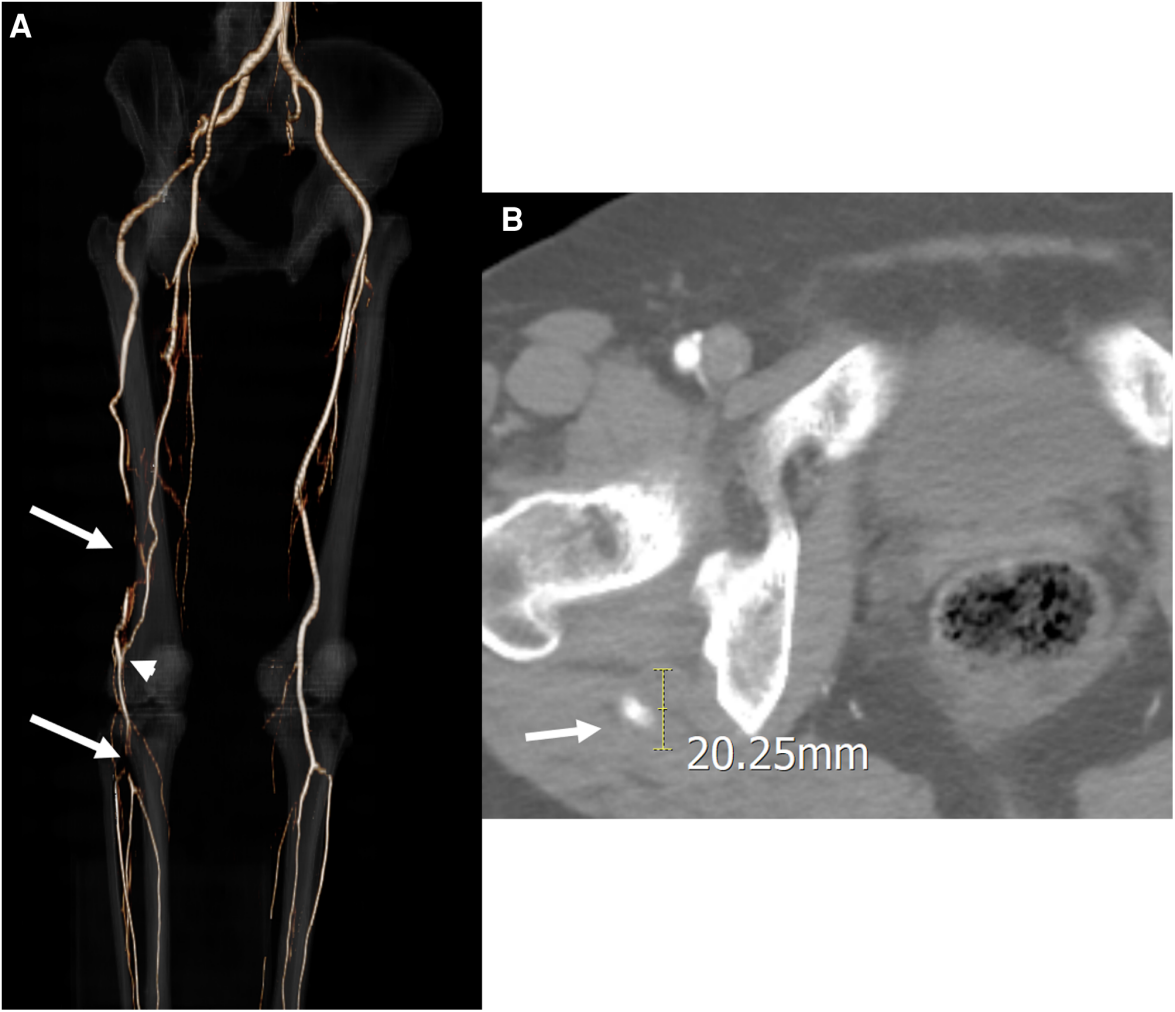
Initial computed tomography angiography (CTA) of the first case. (A) Three-dimensional reconstruction image revealed a persistent sciatic artery (PSA) arising from the right internal iliac artery and two distal thromboembolic occlusions located at the distal PSA in the thigh and the popliteal artery at the trifurcation (*arrows*). A collateral arterial flow was detected between the two thromboembolic occlusions (*arrowhead*). (B) Axial CTA imaging revealed a PSA aneurysm 20 mm in diameter with a mural thrombus near the posterior part of the greater trochanter of the femur.
